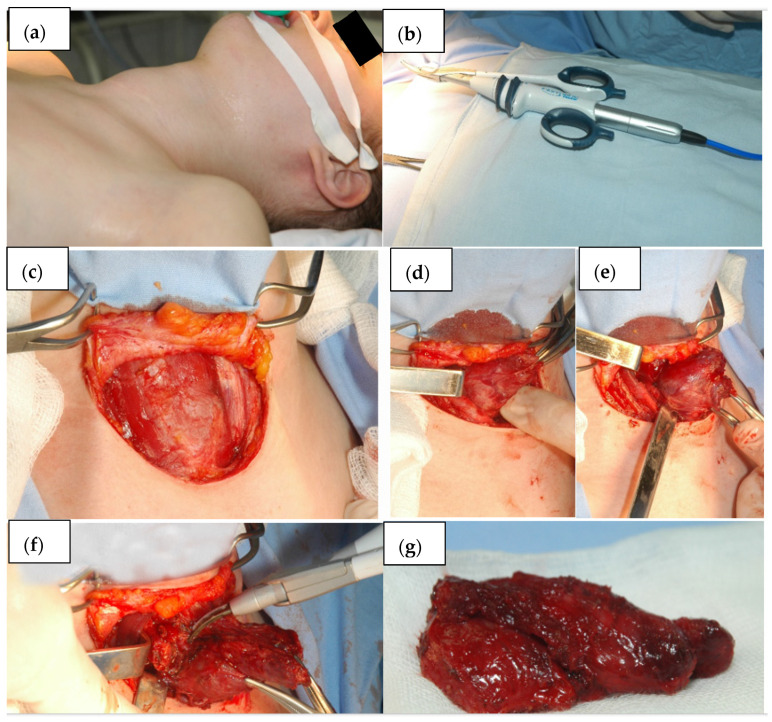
Thyroid lobectomy with isthmusectomy. (a)—Patient before the operation, (b)—ultrasonic surgical device, (c)—upper and lower skin flap is formed under the platysma, (d,e)—mobilisation of the thyroid gland, (f)—ligation and resection of the thyroid artery, (g)—thyroid lobe with isthmus.
